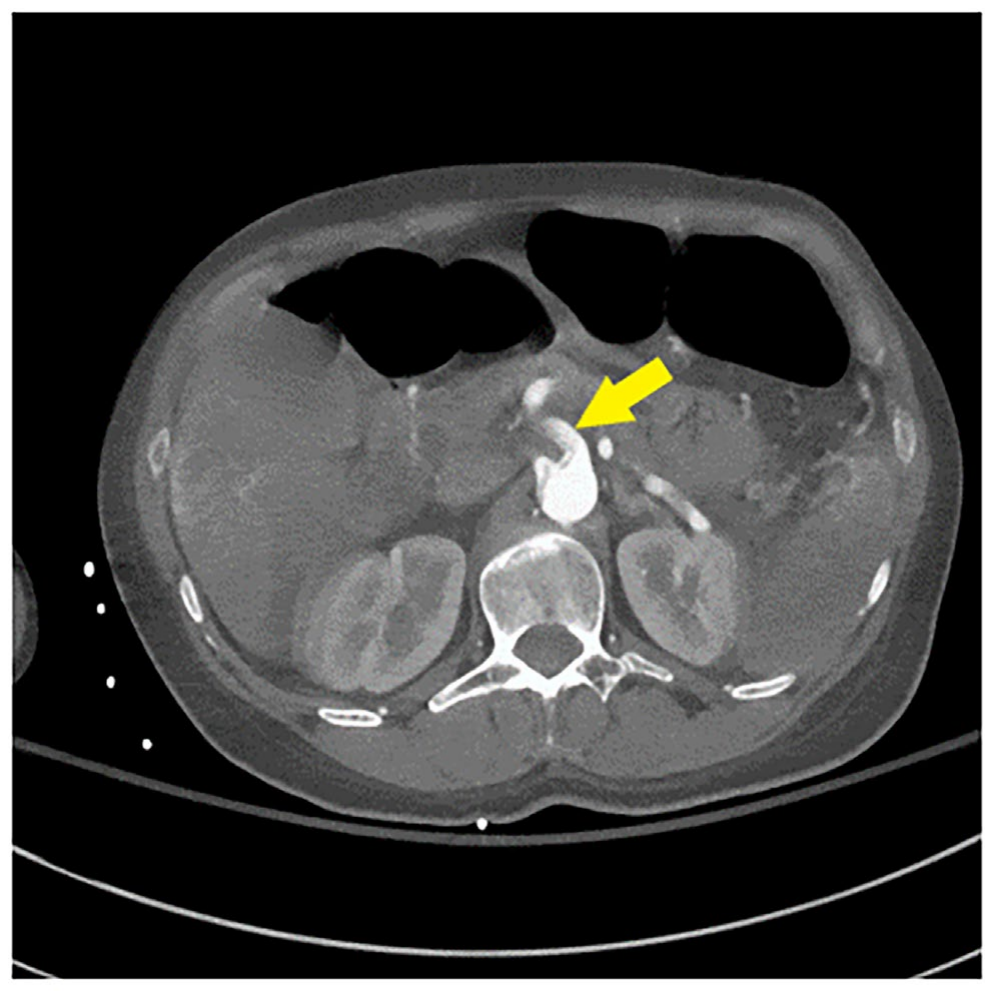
Axial images of CTA of abdomen demonstrates extension of dissection flap extending into the promixal superior mesenteric artery. The celiac, left renal and inferior mesenteric artery all arise from the false lumen. (yellow arrow). Right renal artery arises from the true lumen.
